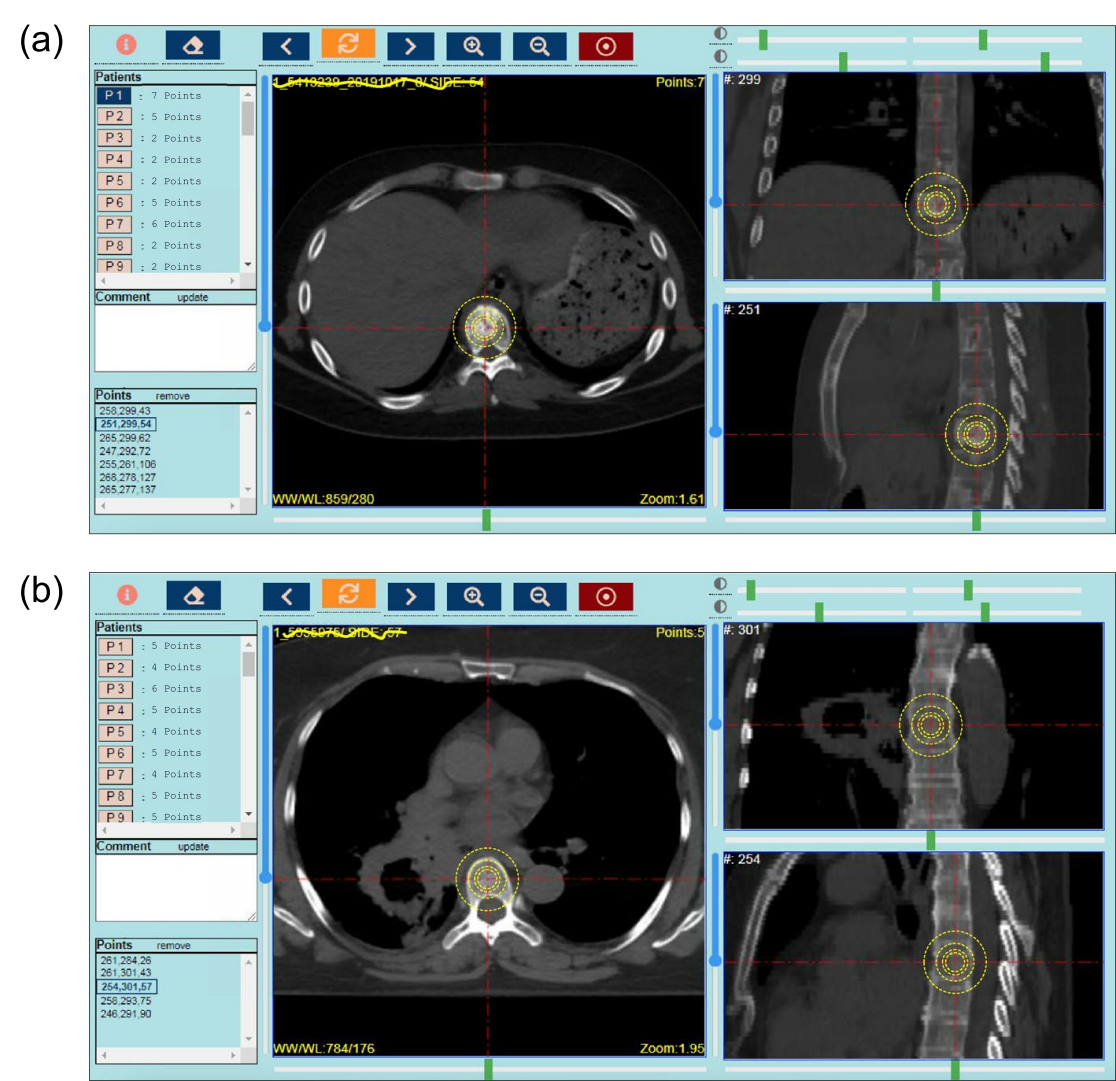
Screenshots of our diCOMBINE 3D lesion labeling web application showing expert-labeled points. (a) A BM lesion, and (b) a HB point. Cross sections of 50 mm, 30 mm, 20 mm, and 15 mm spherical ROIs are visualized with yellow dashed lines on each CT plane.
